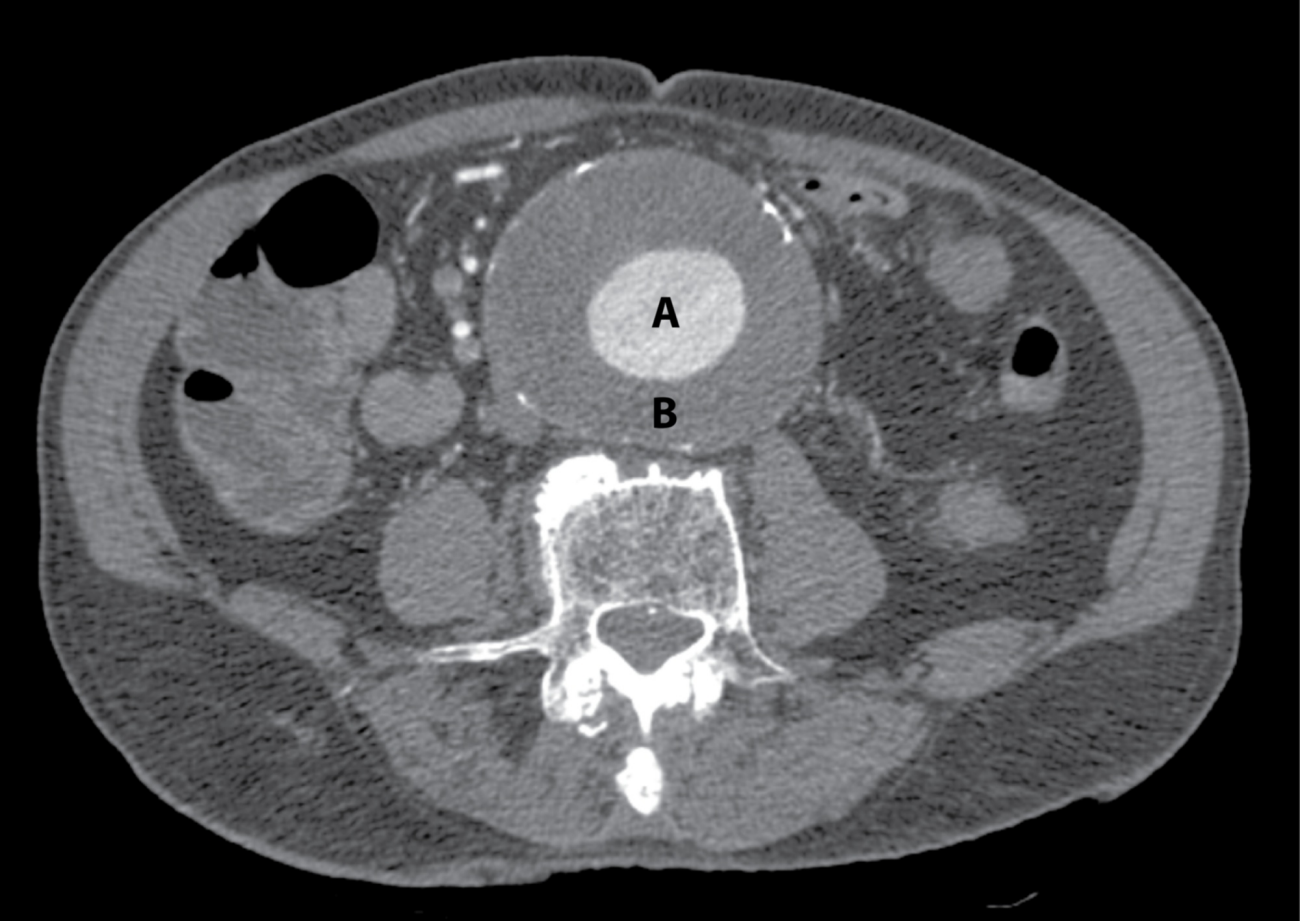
Axial computed tomography scan of the abdomen The axial computed tomography scan showed a large abdominal aortic aneurysm with a central lumen (A) and homogenous mural thrombus (B), with some calcification of the vessel wall. The contrast-enhanced images showed no extravasation or dissection and no additional useful information.
